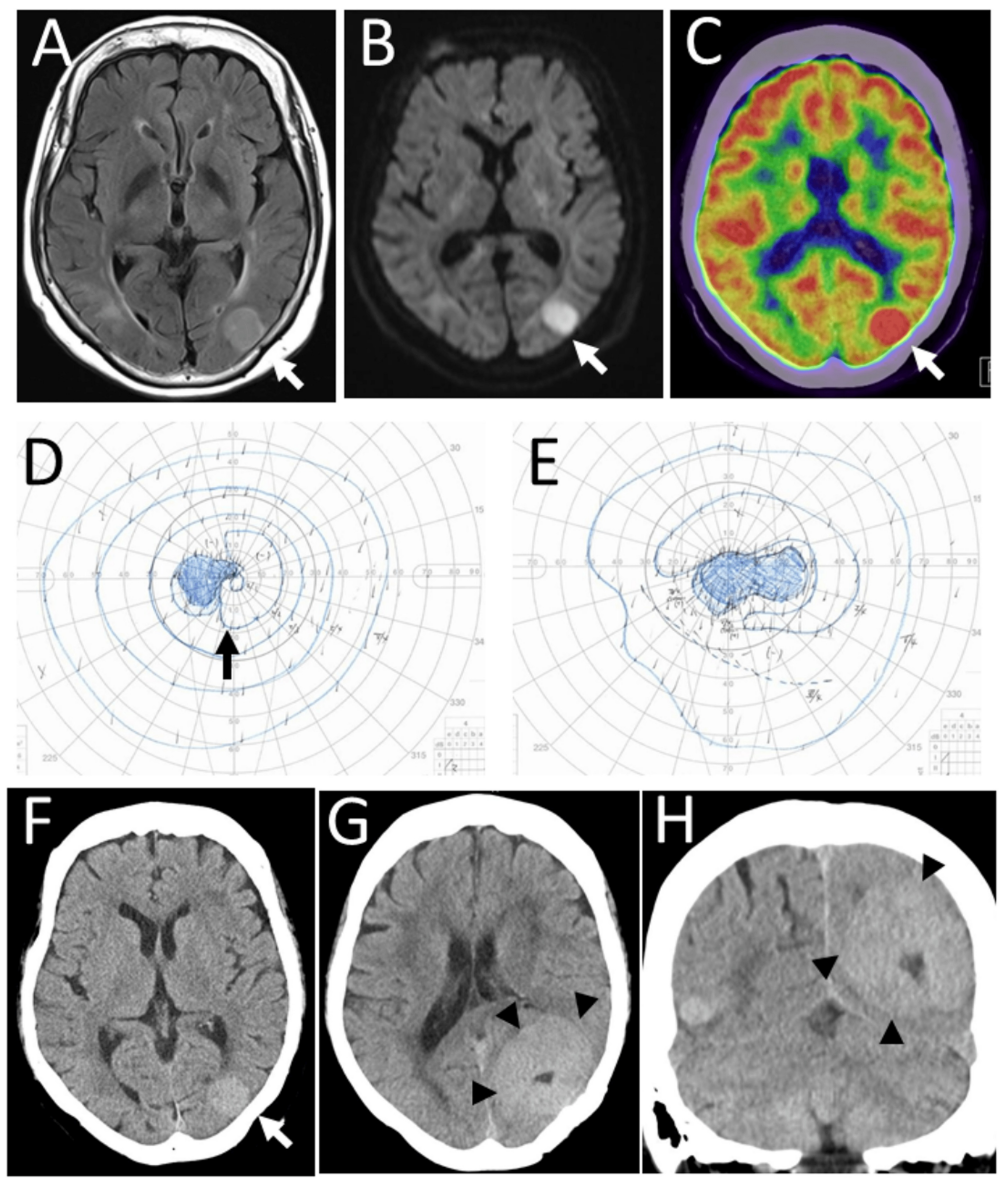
Magnetic resonance imaging, positron emission tomography, Goldmann perimetry, and computed tomography at the age of 76 years Magnetic resonance imaging at the age of 76 years showing a left occipital lesion (arrows) in T2-weighted FLAIR image (A) and diffusion-weighted image (B). Fluorodeoxyglucose positron emission tomography (C) showing high uptake (arrow) in the left occipital lesion. Goldmann perimetry (D: left eye; E: right eye) showing hemianopsia-like change (arrow) in the central vision of the left eye (D) and large central scotoma in the right eye (E). Computed tomography scans: the left occipital lesion (arrow) with no hemorrhage (F) one week after brain biopsy and the enlarged left occipital lesion (arrowheads) half a year later (G: axial image; H: coronal image). FLAIR: fluid-attenuated inversion recovery
